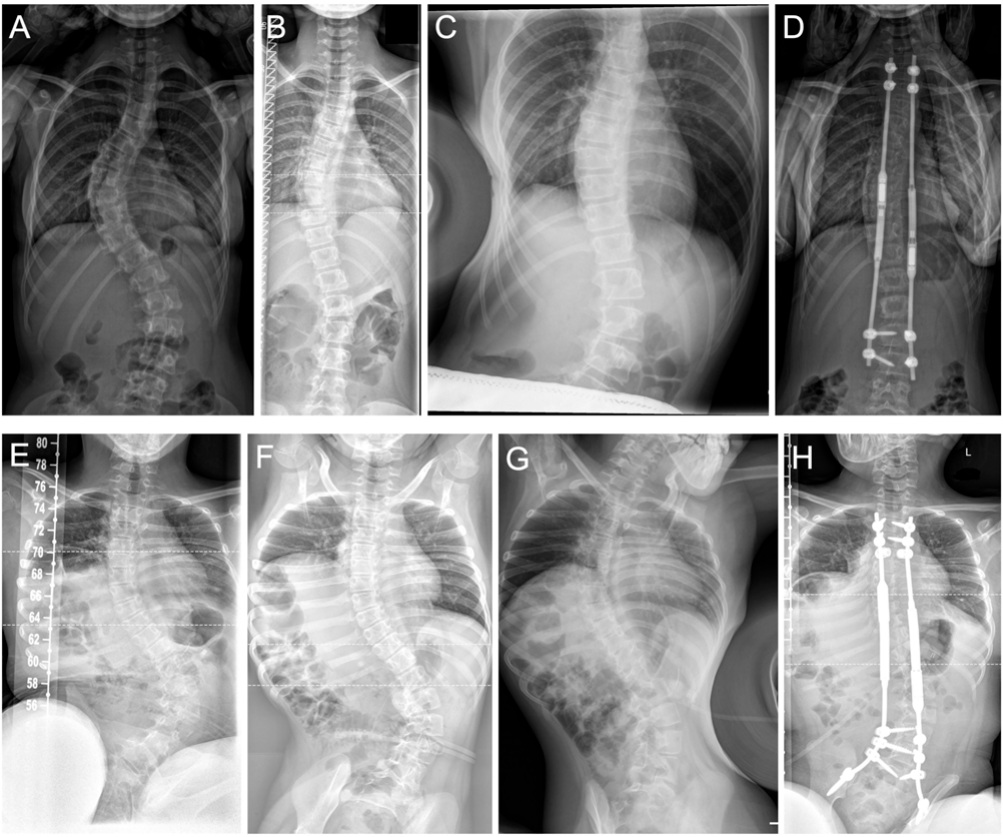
A. p. radiographs showing correction potential of the main curve. Upper panel: Patient suffering from IS: (A) Preoperative standing radiograph. (B) Preoperative supine traction radiograph. (C) Preoperative fulcrum bending radiograph. (D) Postoperative standing radiograph after primary MCGR-implantation. Lower panel: Nonambulatory patient suffering from NMS: (E) Preoperative sitting radiograph. (F) Preoperative supine traction radiograph. (G) Preoperative fulcrum bending radiograph. (H) Postoperative sitting radiograph after primary MCGR-implantation.
